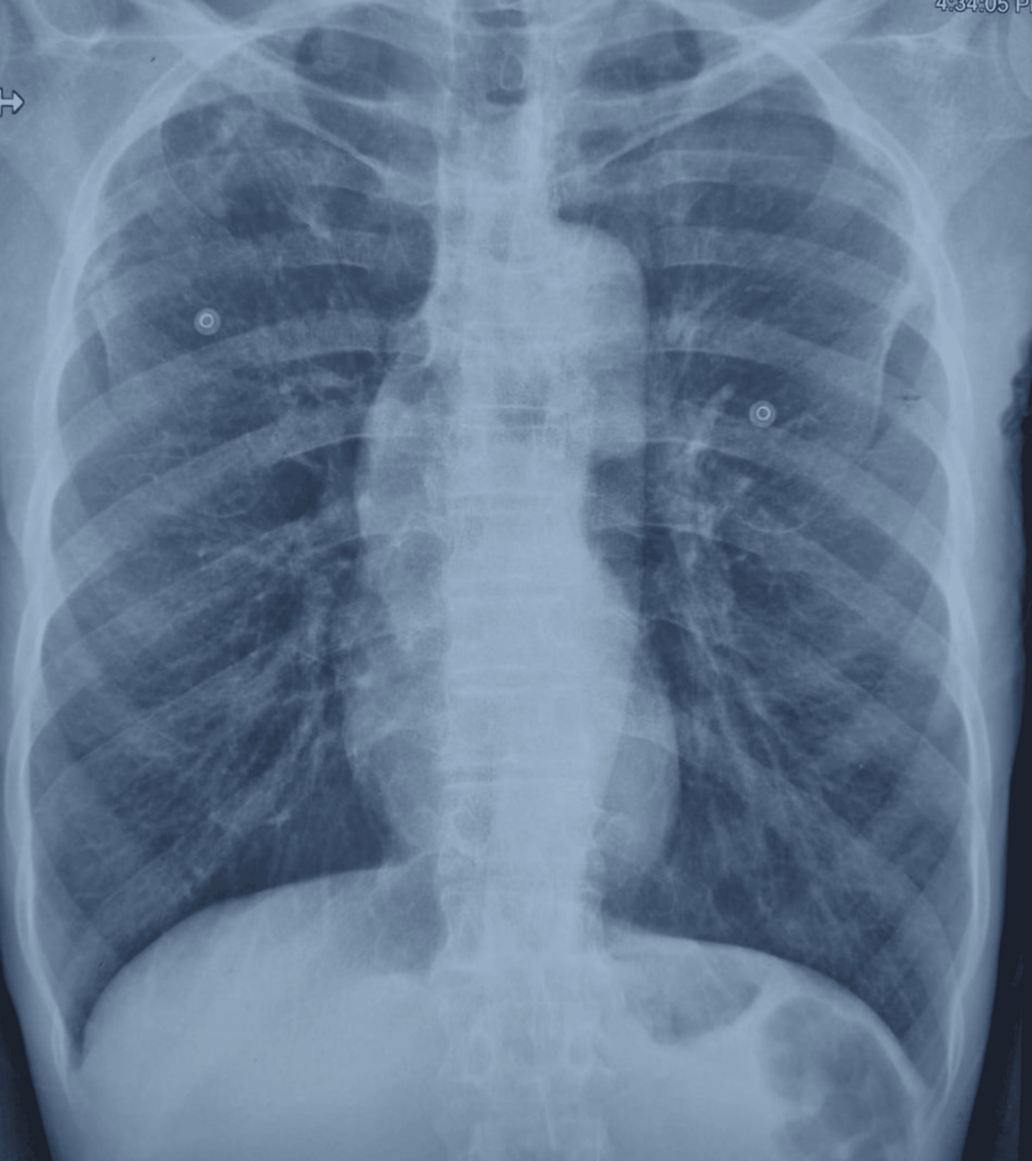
Anterior-posterior view of chest X-ray of the patient with normal bony structures, airway passages, lung parenchyma, and cardiac shadow.
